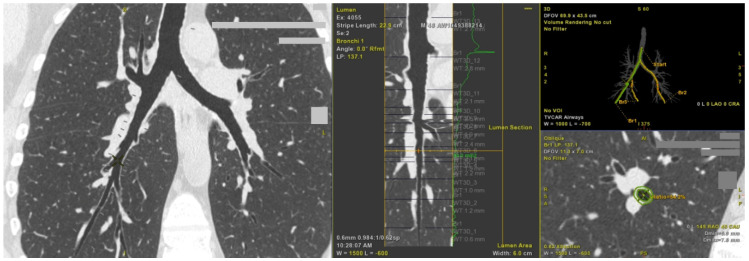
Lung computed tomography—automated measurements of airway geometry parameters in the right lower lobe basal posterior bronchus (RB10).
